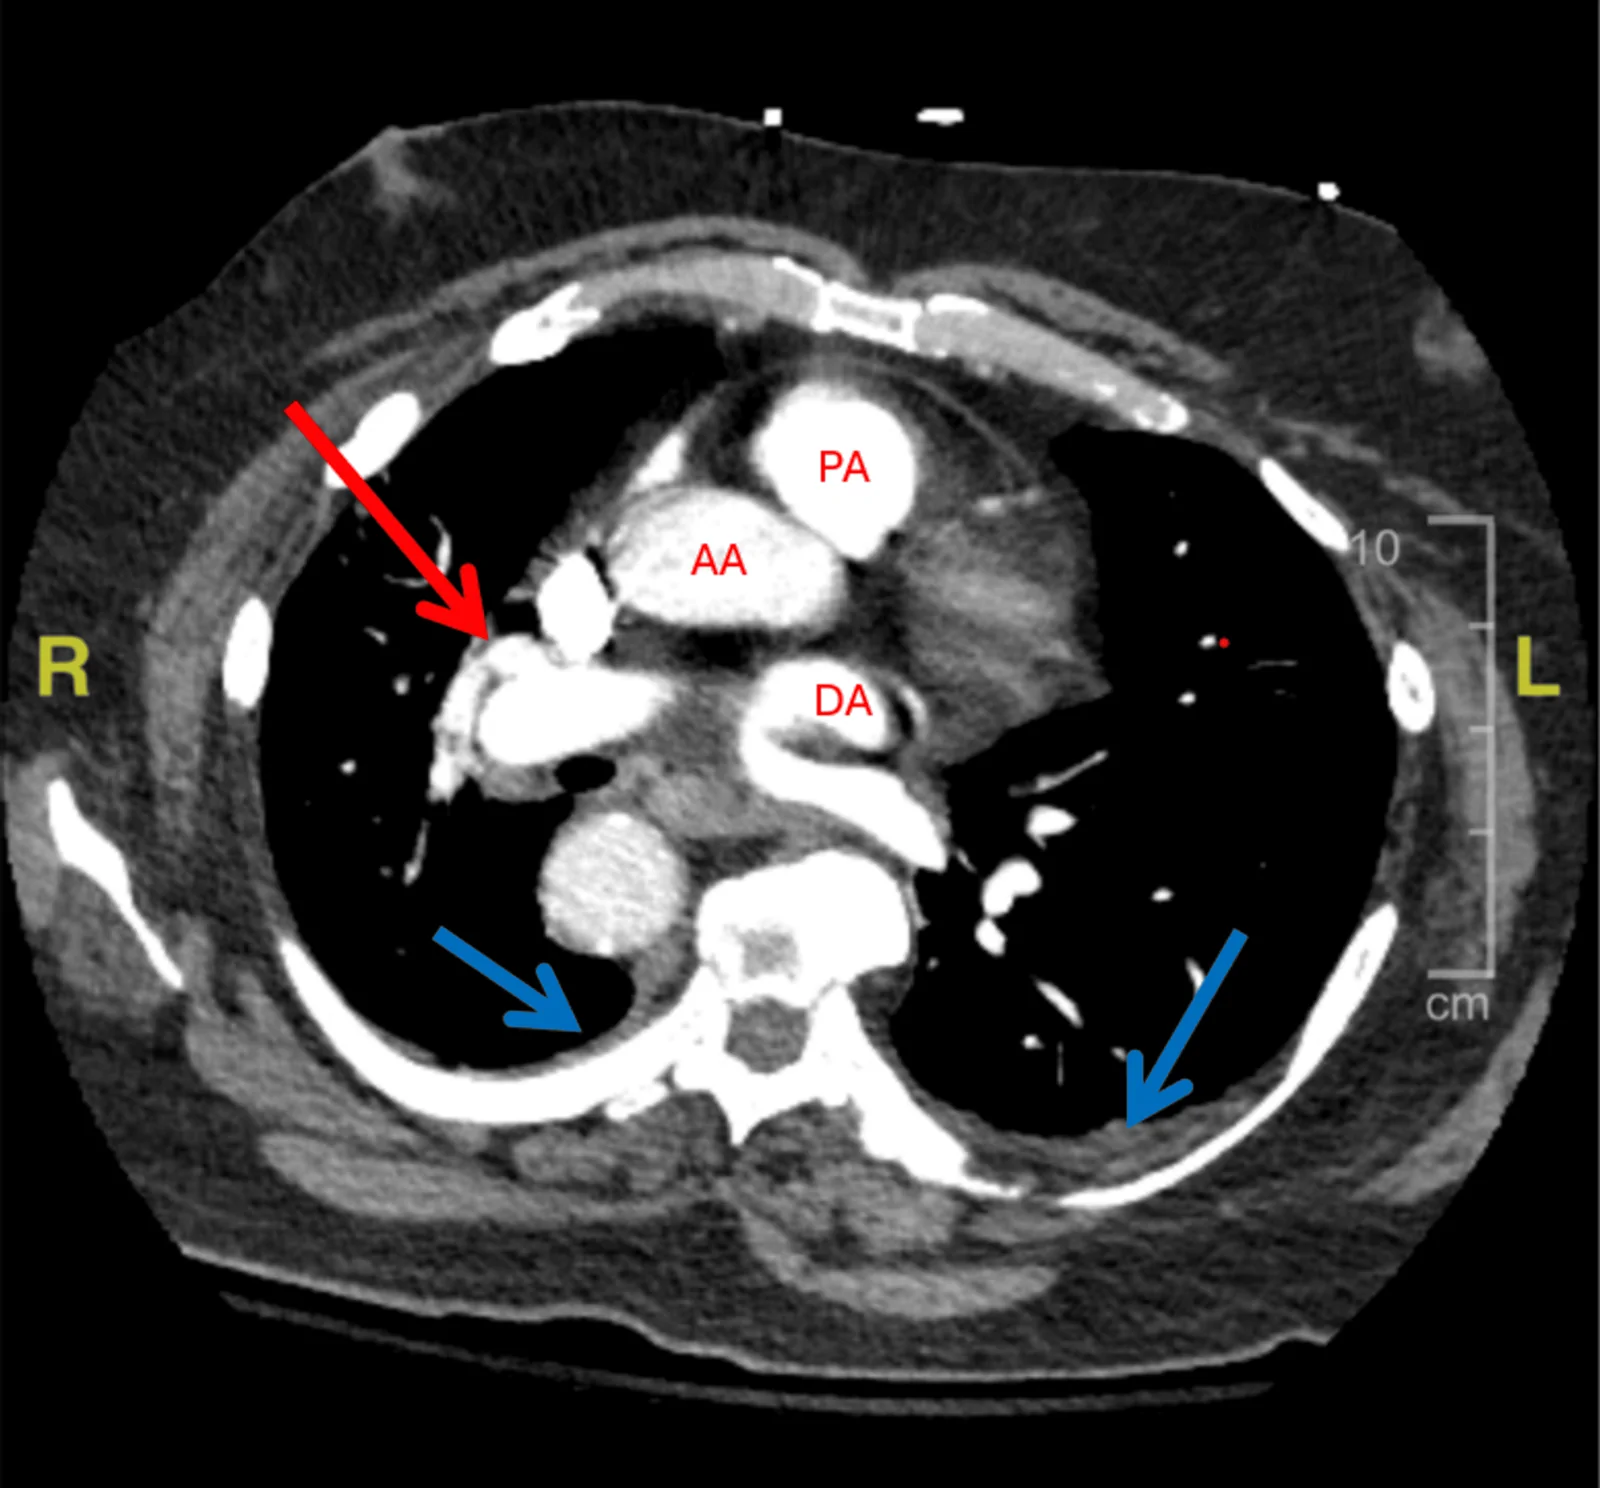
CTA of the neck There are moderate-sized bilateral pleural effusions (blue arrows) and bilateral airspace opacities. The Kommerell diverticulum measures approximately 4.0 cm (red arrow), a size associated with an increased risk of compressive symptoms and warranting vascular surgery evaluation. CTA: computed tomography angiography; AA: abdominal aorta; PA: pulmonary artery; DA: descending aorta; R: right; L: left
